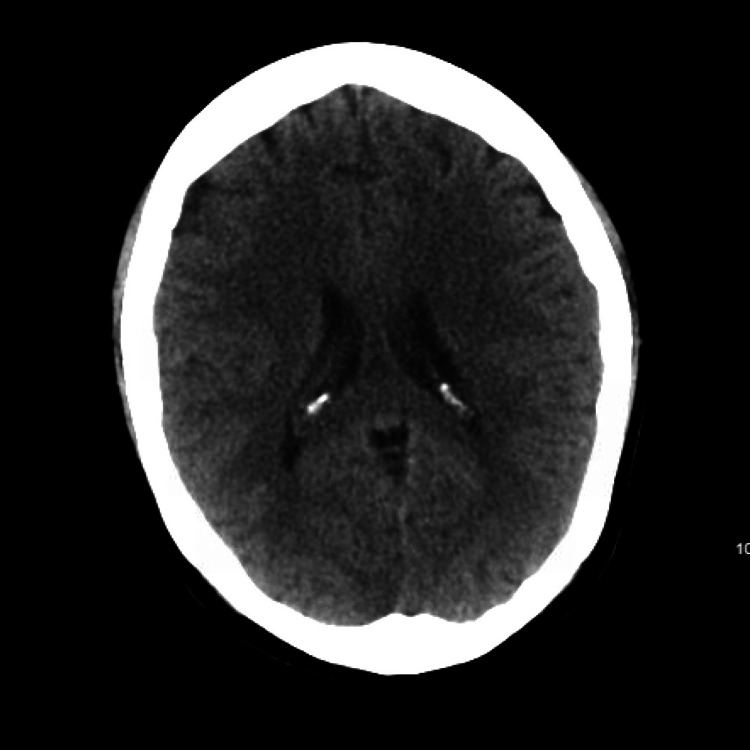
CT head obtained during the rapid response showing no acute abnormality of the brain parenchyma CT, computed tomography
